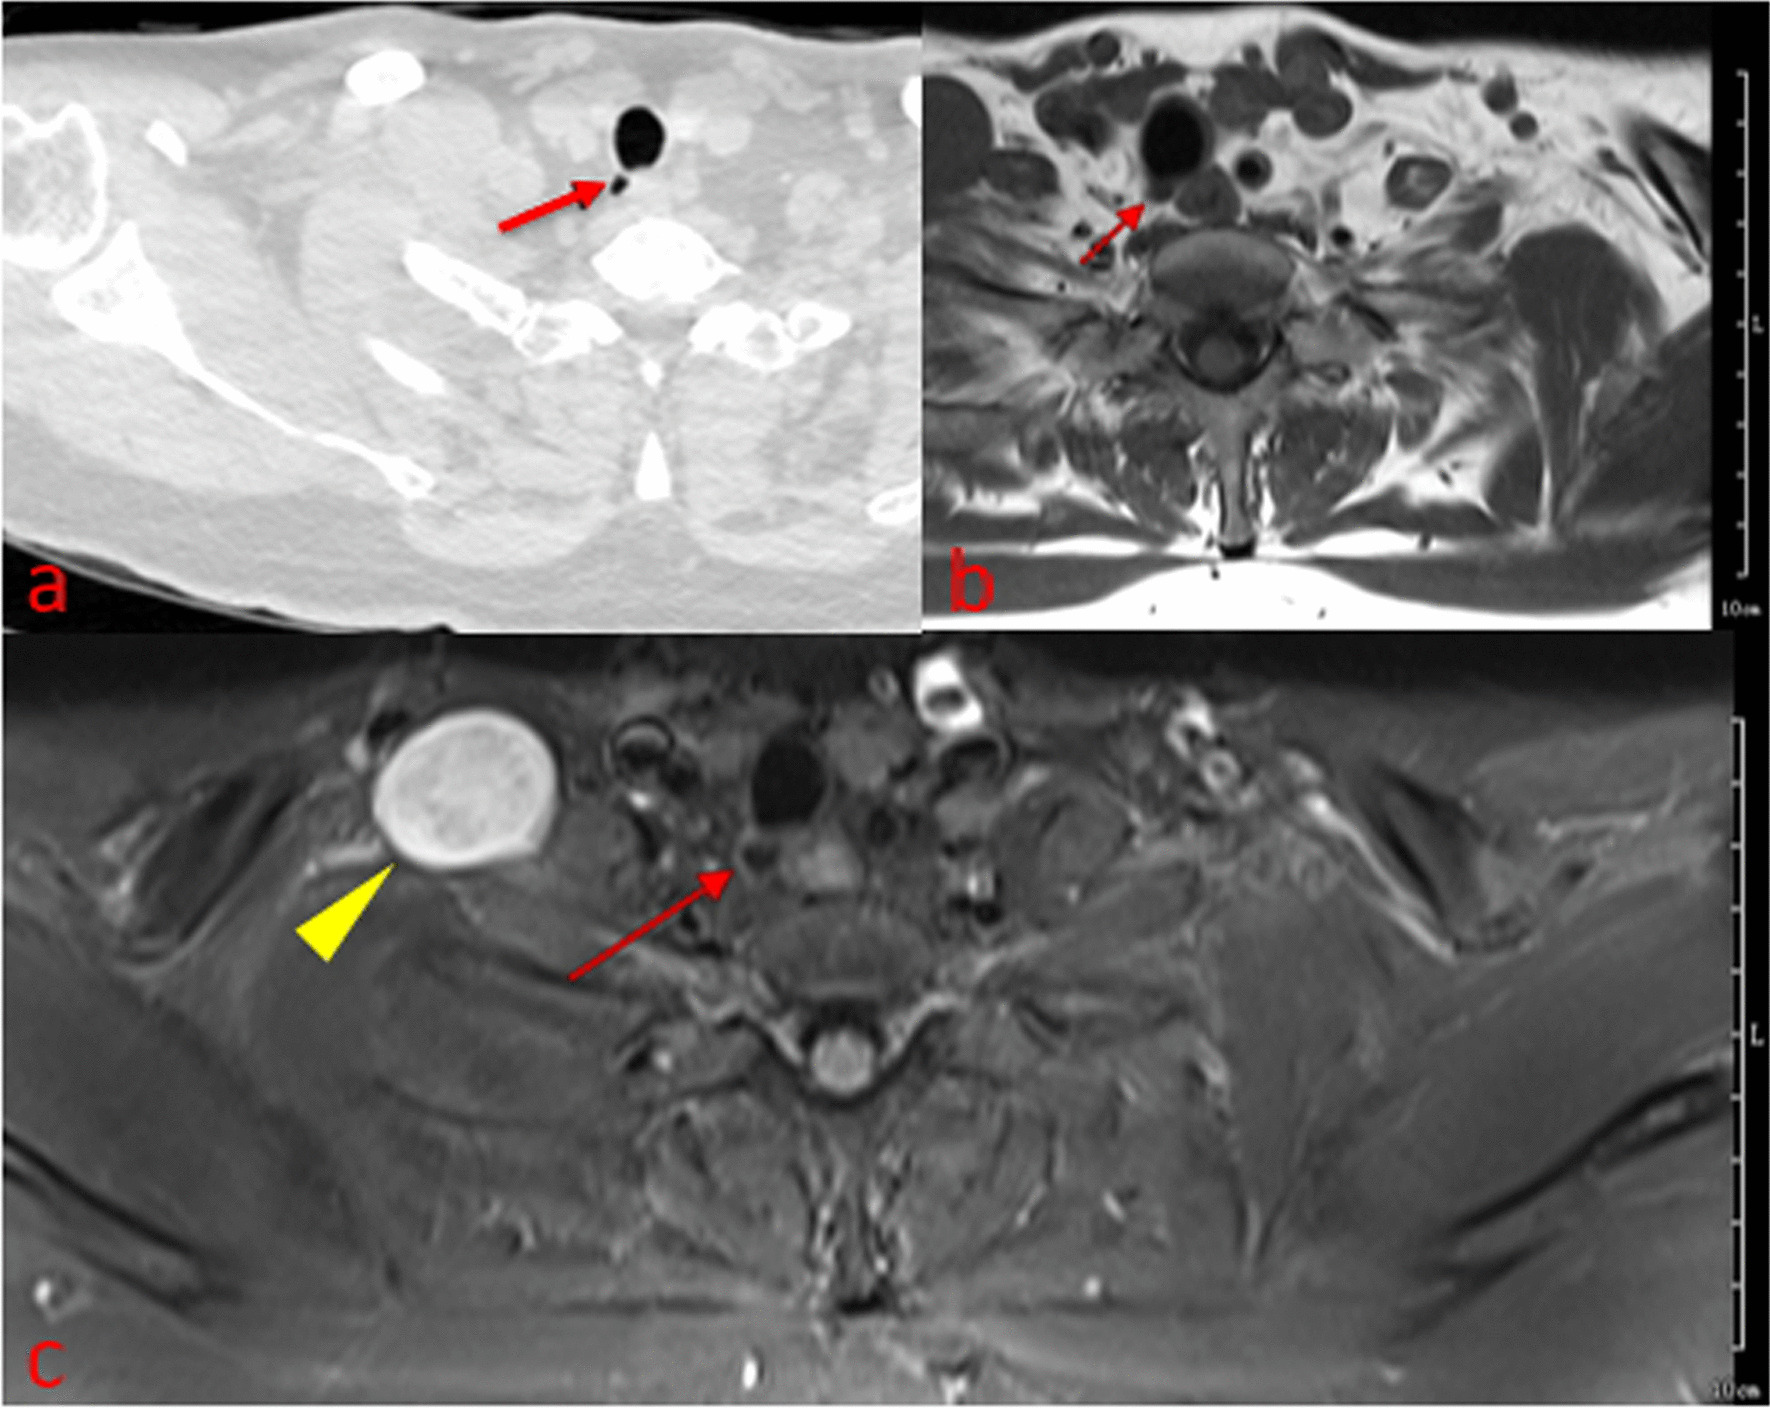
Axial CT image (a) in lung window showing a 5-mm diverticulum along the right posterolateral wall of the upper trachea (arrow). Axial T1WI (b) and T2-TIRM (c) showed a circular air-filled cystic lesion (no signal area) just behind the right side of the trachea (arrow). T2-Tirm clearly showed the slightly high signal wall of the lesion. Communication between the air cyst and the trachea was not seen in CT or MR images. A schwannoma in the right supraclavicular fossa is shown (arrowhead)
